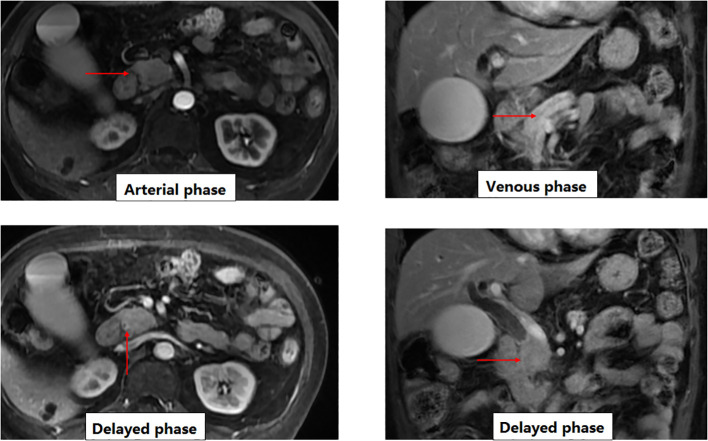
Dynamic contrast-enhanced MRI findings of the distal common bile duct and pancreatic head show a soft tissue mass at the distal common bile duct (red arrow), exhibiting relatively low enhancement in the arterial phase. In the venous phase, the lesion demonstrates moderate, gradual enhancement (red arrow). During the delayed phase, the lesion shows persistent and progressive enhancement (red arrows), a radiologic pattern that may be seen in fibroinflammatory lesions such as IgG4-related sclerosing cholangitis and that, in this case, supported further tissue-based evaluation. Although these findings did not exclude malignancy, the delayed progressive enhancement pattern raised the possibility of a fibroinflammatory process and supported further tissue-based evaluation.
